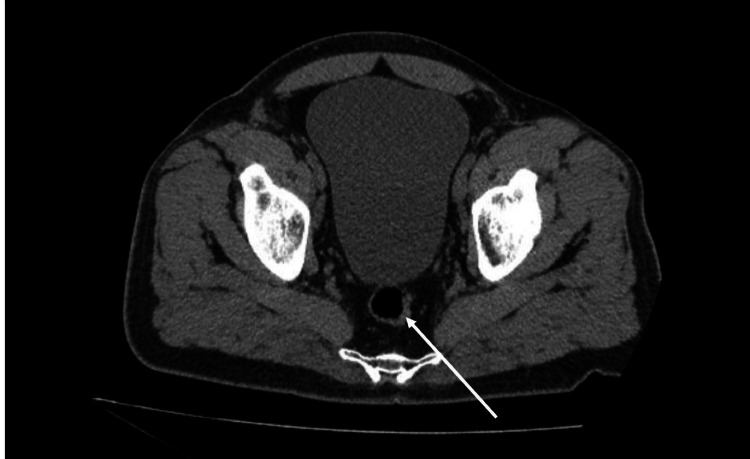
CT scan of the abdomen and pelvis showing the perianal abscess (white arrow). CT, computed tomography
